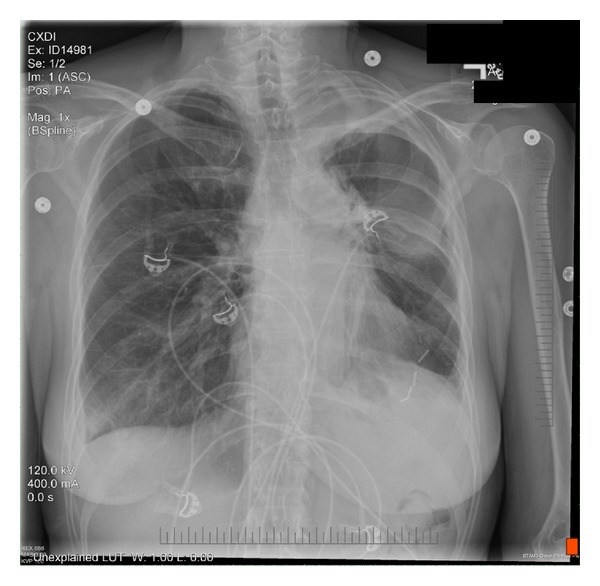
Posterior to anterior chest X-ray shows left perihilar parenchymal scars, surgical clips, and postoperative changes in the chest.
